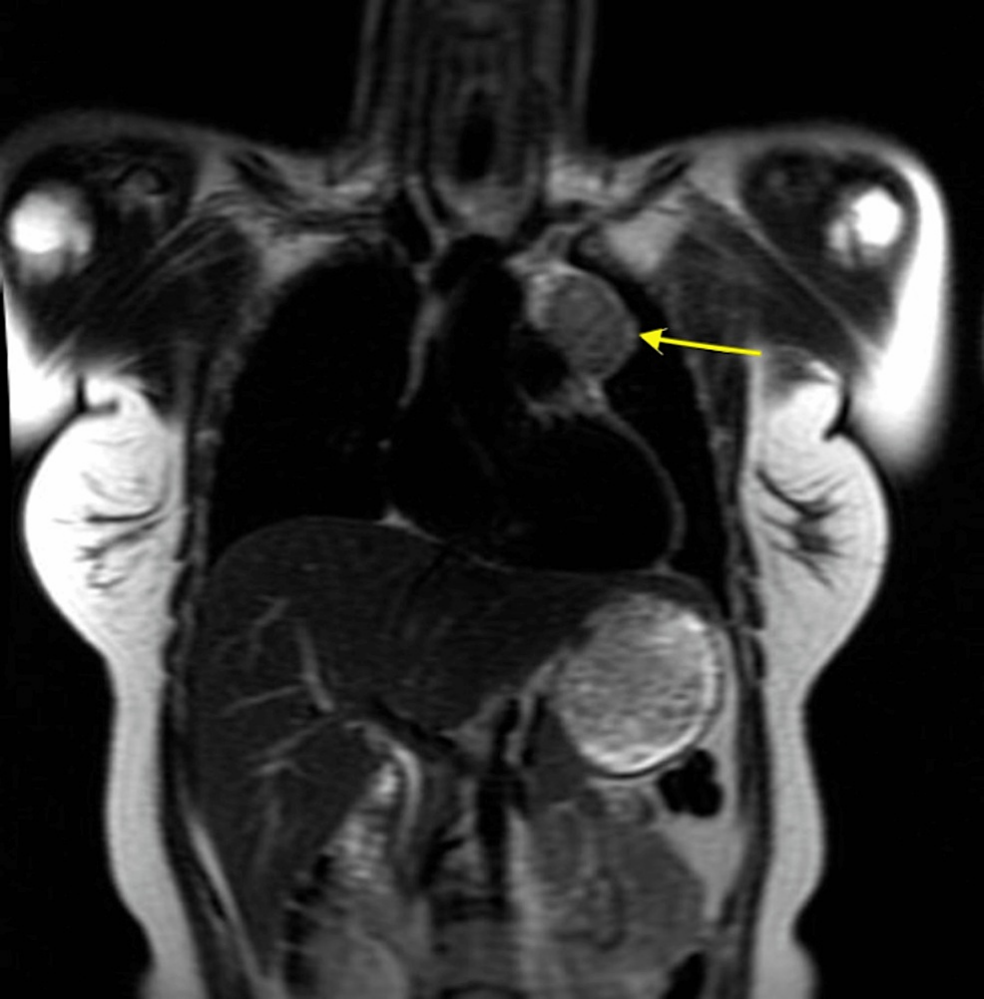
MRI thorax coronal view showing a thymoma. MRI: magnetic resonance imaging.
